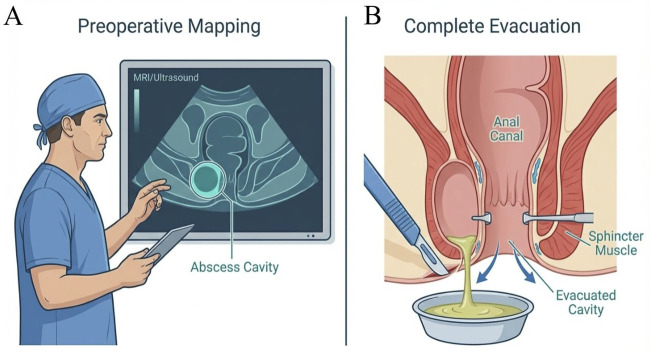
Optimization of incision and drainage (I&D) in pediatric perianal abscesses. (A) Preoperative mapping using magnetic resonance imaging (MRI) or ultrasound enables precise delineation of abscess boundaries and their spatial relationship to the anal sphincter complex. (B) Surgical execution prioritizes complete evacuation of the abscess cavity through a minimal incision, while strictly preserving the integrity of the internal and external anal sphincter muscles. This anatomy-guided approach minimizes iatrogenic sphincter injury during the acute inflammatory phase.
